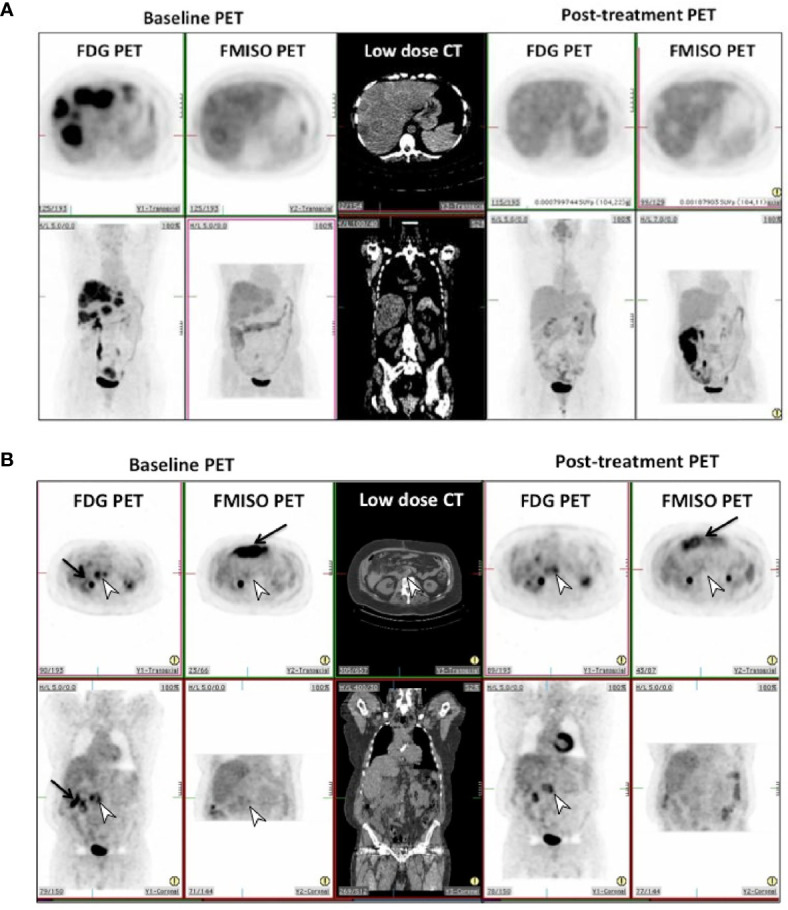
(A) Pre and post-treatment FDG and FMISO PET scans in patient 8 in transverse (top row) and coronal (bottom row) projections. This shows complete metabolic and hypoxic response in the liver metastases to treatment, with photopenic defects in the liver on post-treatment scans. (B) Pre and post-treatment FDG and FMISO PET scans in patient 12 in transverse (top row) and coronal (bottom row) projections. This shows incomplete metabolic and hypoxic response to treatment on FDG & FMISO PET in retroperitoneal lymph nodes seen on CT (white arrowhead). Intense bowel activity on FMISO scans is noted anteriorly (black arrows).
